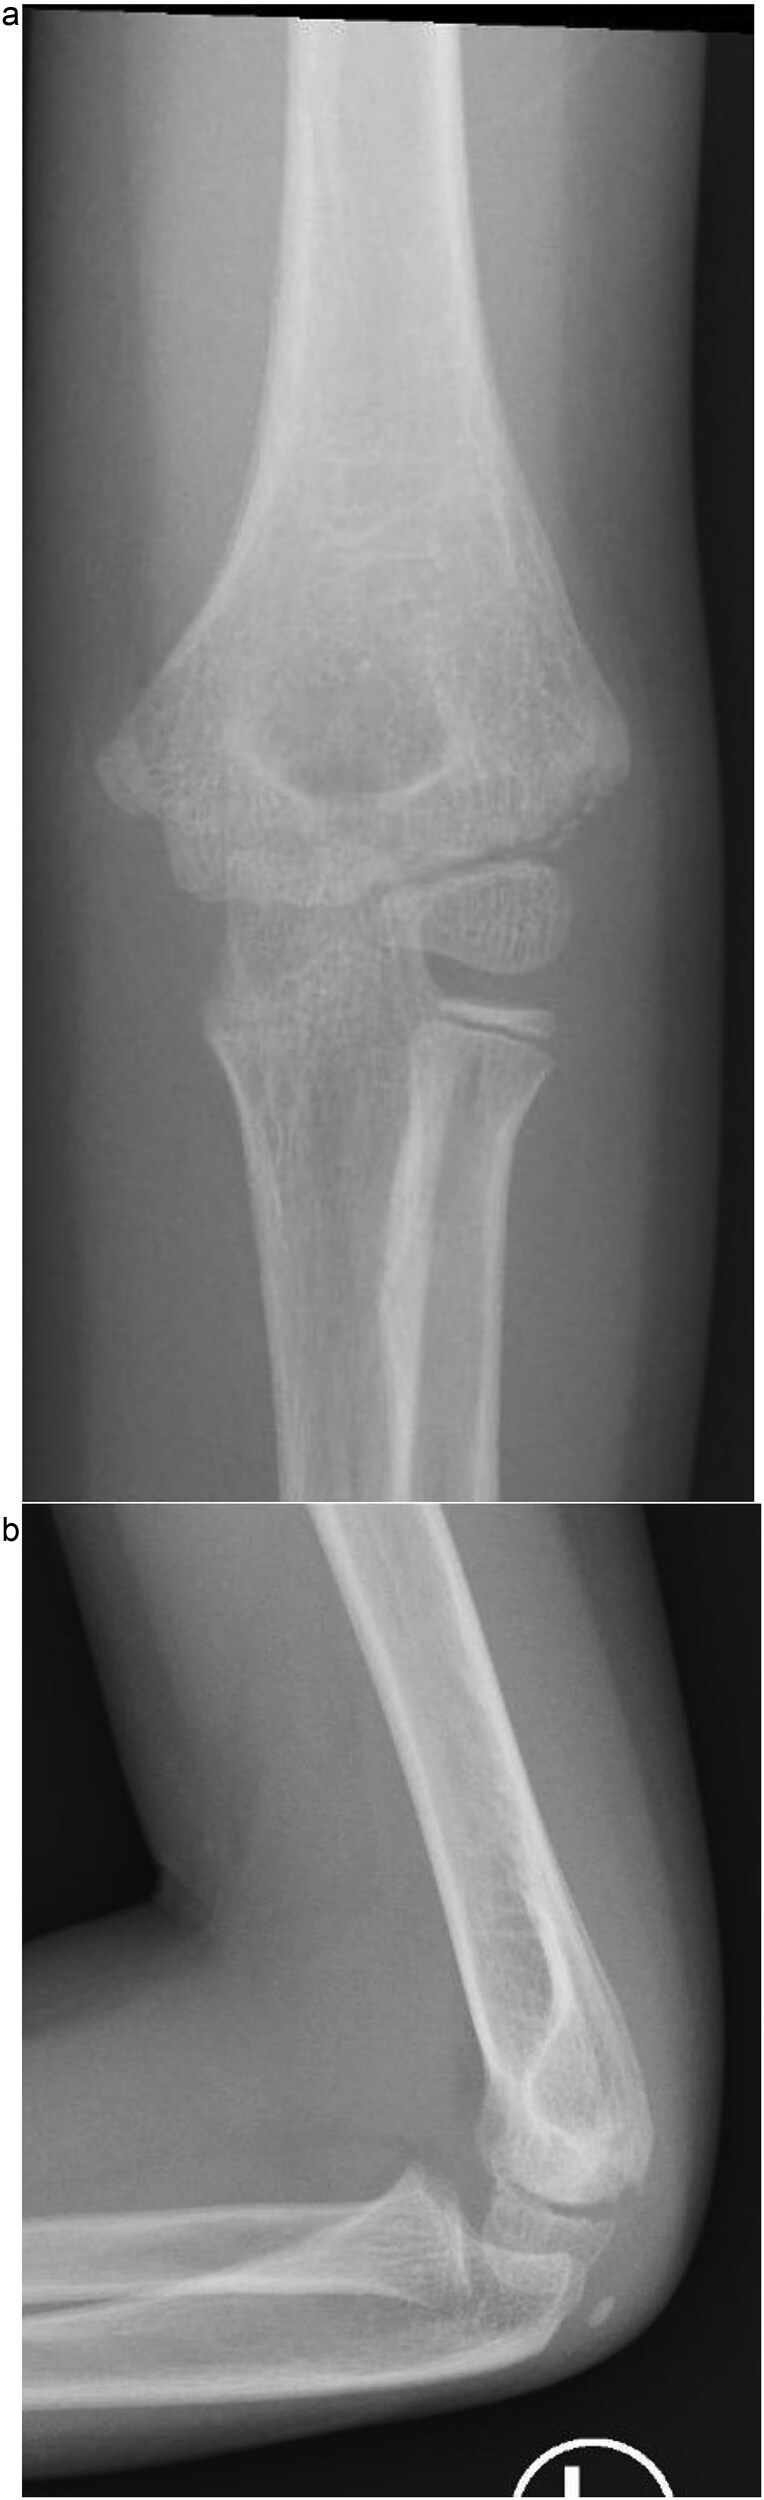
(a) Immediate post-reduction anteroposterior radiograph showing restored joint alignment with persistent lateral condyle displacement. (b) Lateral view confirming maintained reduction of the ulnohumeral joint and residual separation of the lateral condyle fragment.
